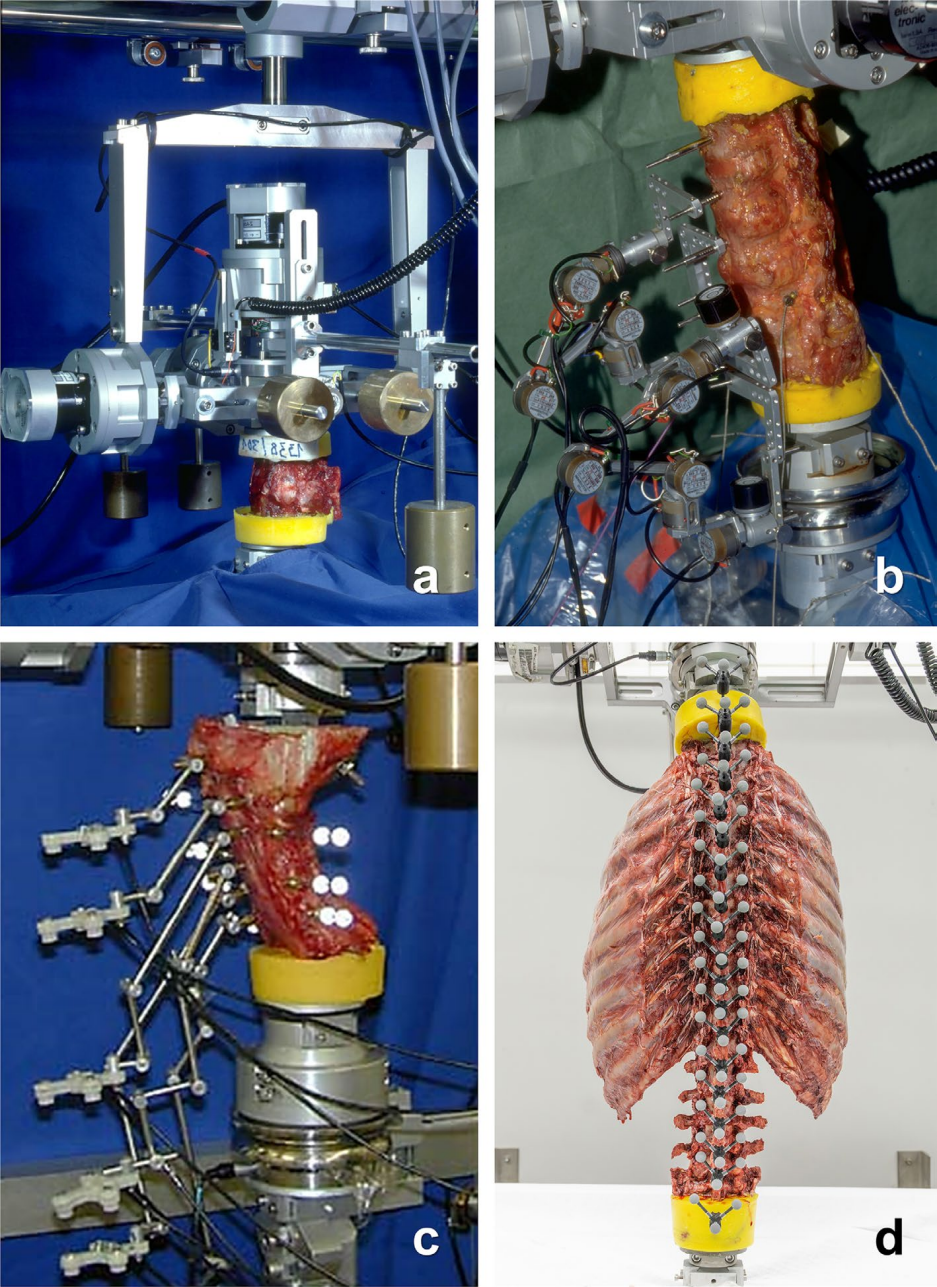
Measurement systems used in studies at the authors' institution between 1995 and 2024: Rotary variable displacement transducers integrated into the cardan joint of the spine tester (a), three‐dimensional goniometric linkage system (b), ultrasound‐based motion analysis system Zebris (c), optical motion tracking system Vicon MX13 (d).
